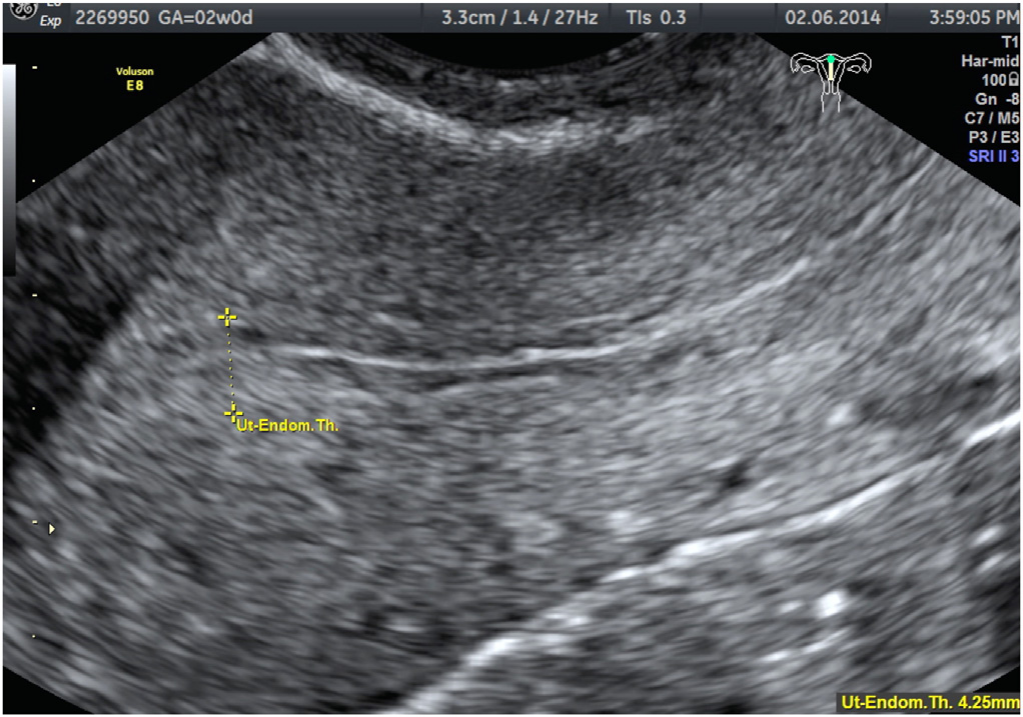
Ultrasound of uterus demonstrating empty uterine cavity and normal endometrial thickness. Reprinted from content created by Alexandra Stanislavsky from Radiopaedia.org, rID:47667, under CC BT-NC_SA 3.0 license (https://creativecommons.org/licenses/by-nc-sa/3.0).
